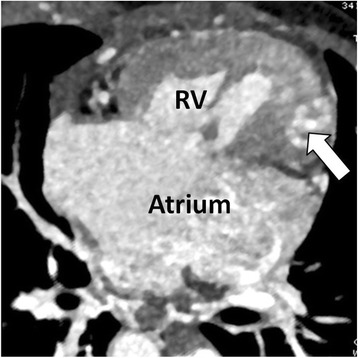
Cardiac CT of a 2 days old infant showing single atrium (Atrium), univentricular right ventricle (RV) and rudimentary left ventricle (arrow)
